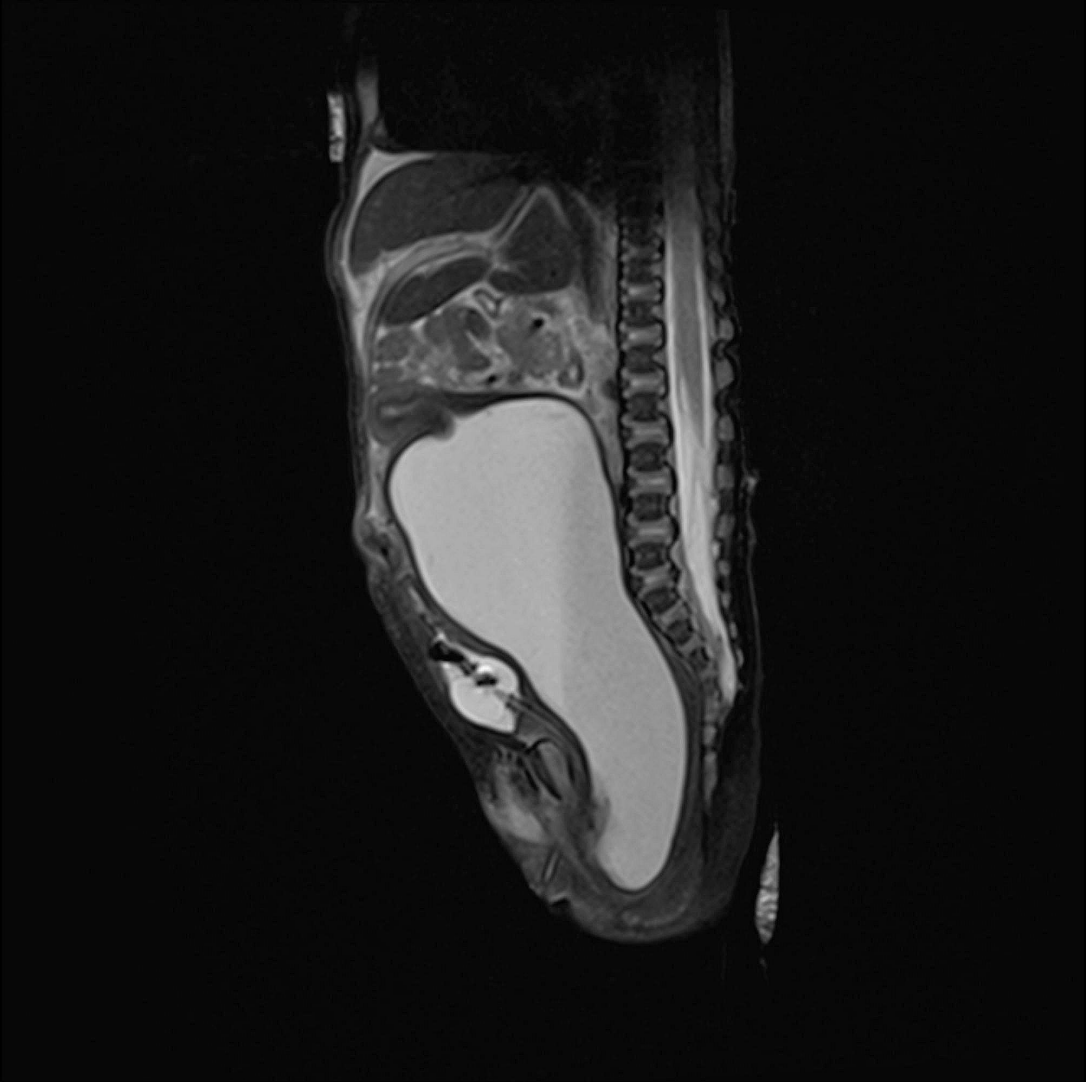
MRI of the abdomen and pelvis. Findings suggested a voluminous vagina with a fluid–fluid level extending from the perineum to the supraumbilical region
